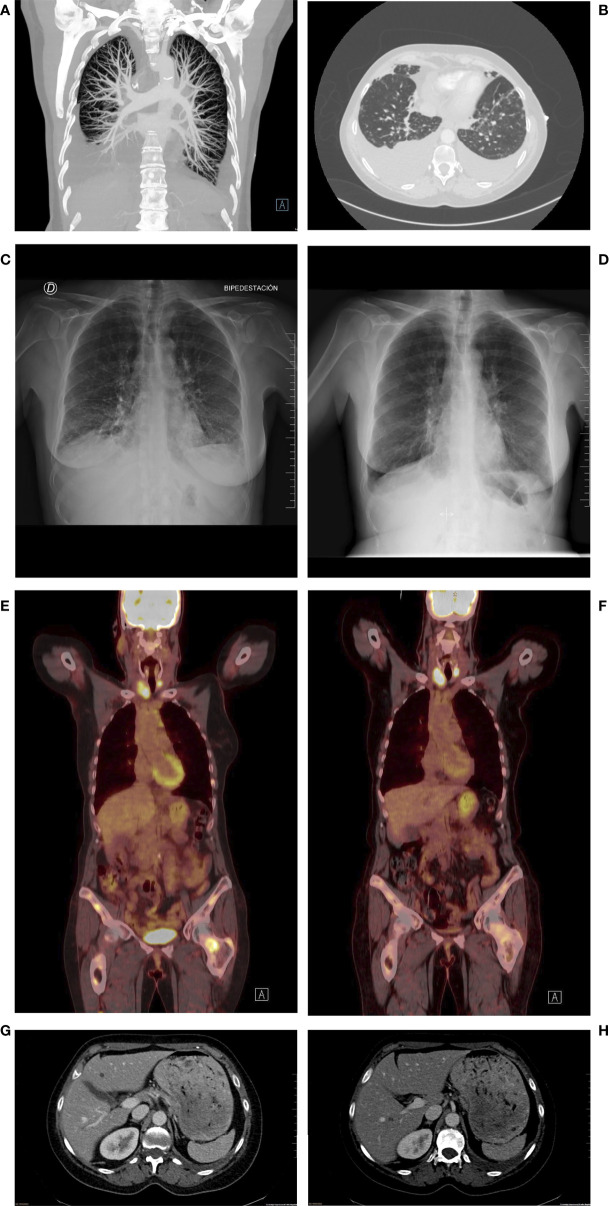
Representative images from the most relevant radiologic assessments. (A, B) CT Angiography at hospitalization showing signs of lymphangitis carcinomatosis. (C, D)Chest X-ray pre (C) and post (D) hospitalization showing the disappearance of lymphangitis signs and considerable reduction in pleural effusion. (E, F): PET/CT imaging at metastatic relapse diagnosis (E) and at first reassessment (F) after first-line treatment start, showing SUV reduction in metastatic sites. (G, H): CT scan at progression, showing two new hepatic lesions in the IV (G) and VI (H) segments.
